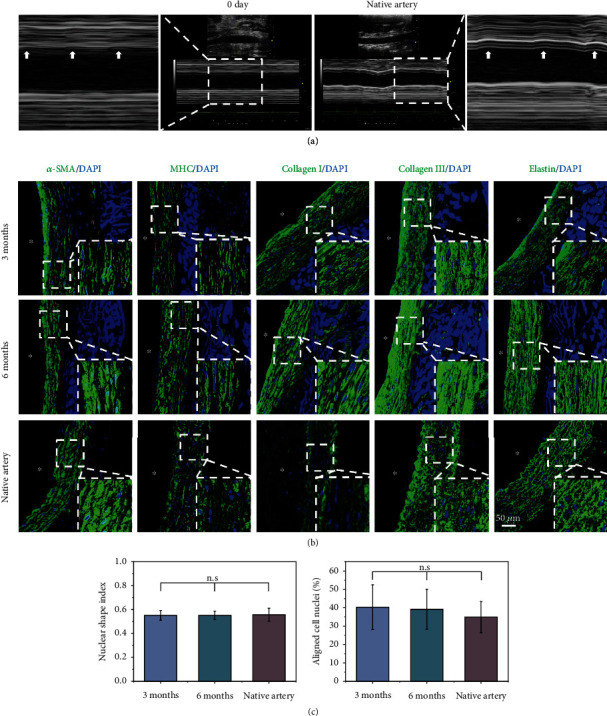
VSMC regeneration and ECM deposition with circumferential orientation *in vivo*. (a) M-mode color Doppler images of vascular grafts 0 days after implantation and the native arteries. (b) VSMC regeneration with circumferential orientation and ECM organization within the graft. (c) NSI and percentage of aligned cells of the native artery and the regenerated grafts. Data are presented as the mean ± SD (*n* = 4). “n.s.” means no significance. Arrows indicate the movement of the inner diameter of regenerated grafts and native artery, which suggests that regenerated grafts pulse synchronously with the native artery. Lumen is marked with ^∗^.
